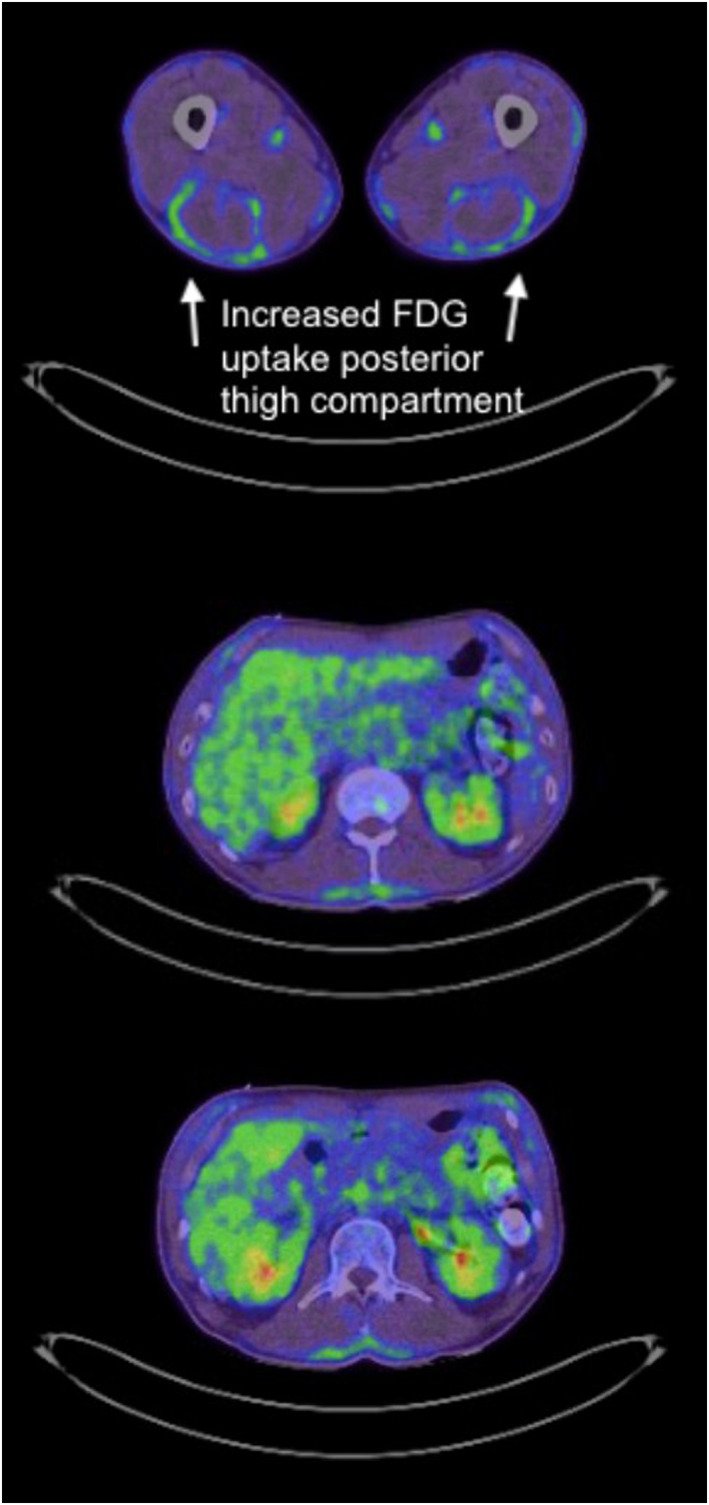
(a–c) PET scan of thighs and trunk showing diffuse increased fascial FDG uptake.
